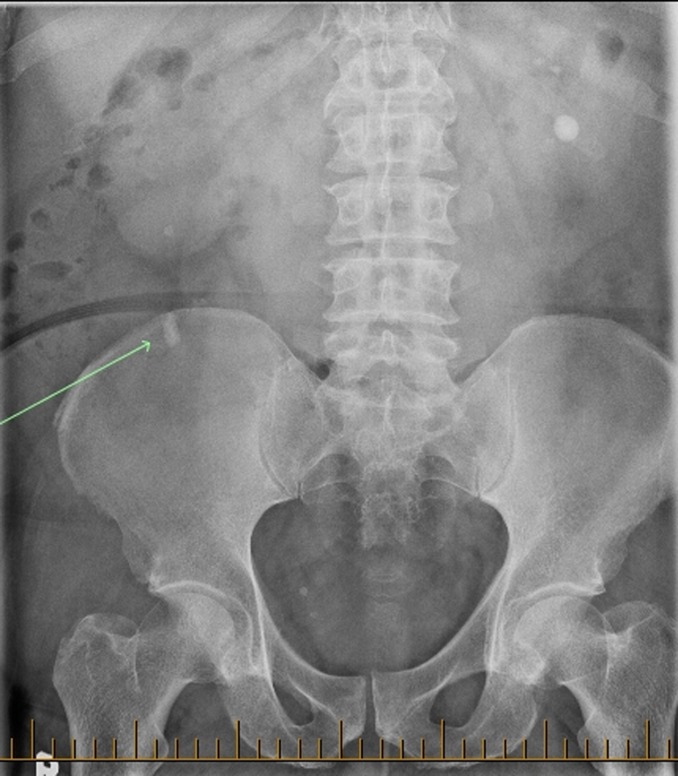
Initial KUB with a right iliac crest calcification (*arrow*) and a left lower pole calcification. KUB = kidney, ureter, and bladder radiograph.
